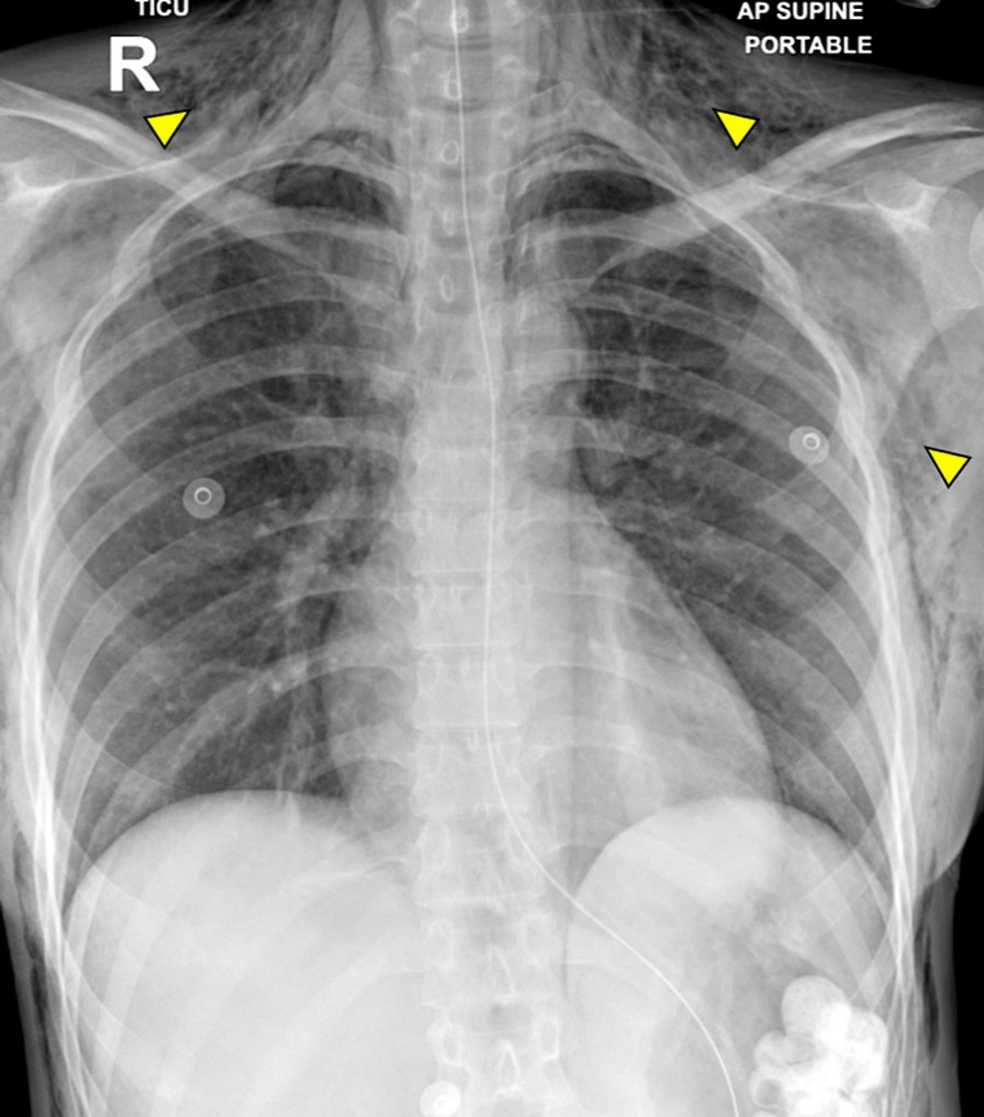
Chest X-ray showing significant resolution of the bilateral pneumothorax with residual subcutaneous emphysema (yellow arrow).
